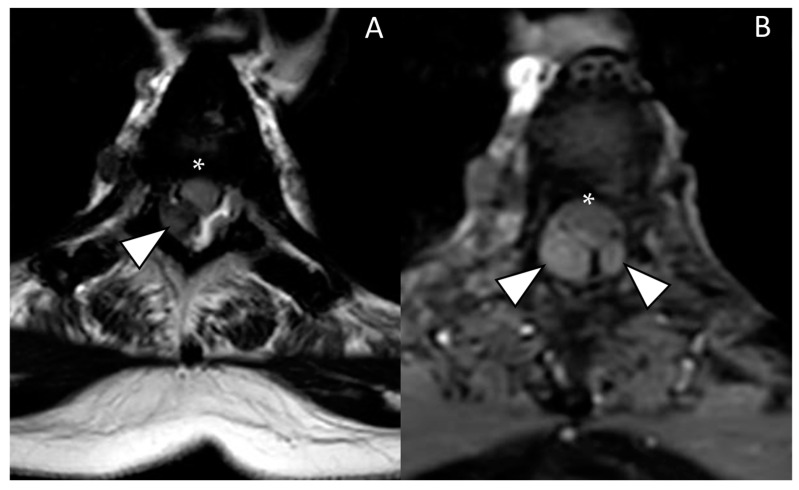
(A) Axial magnetic resonance T1-weighted image; (B) axial magnetic resonance T1 fat-suppressed weighted image after endovenous contrast media administration: axial images show the malignant solid nodules (white arrowheads) on the epidural lipomatosis that compress the spinal cord (white asterisk). Note also the diffuse pathological signal of the vertebral bone marrow.
